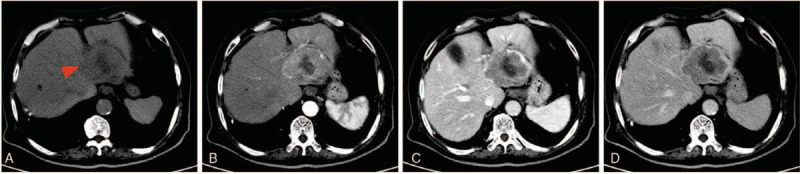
Enhanced CT images of primary hepatic neuroendocrine carcinomas. The enhanced CT demonstrated a “rapid enhancement and rapid washout” pattern. (A) A 6 × 5 cm hypodense lesion with necrosis in the center of the left lobe of the liver (red arrow). (B) The lesion showed rapid enhancement in the arterial phase, and the relatively lower density that followed indicates a quick washout in the portal phase (C) and delayed phase (D). CT = computed tomography.
